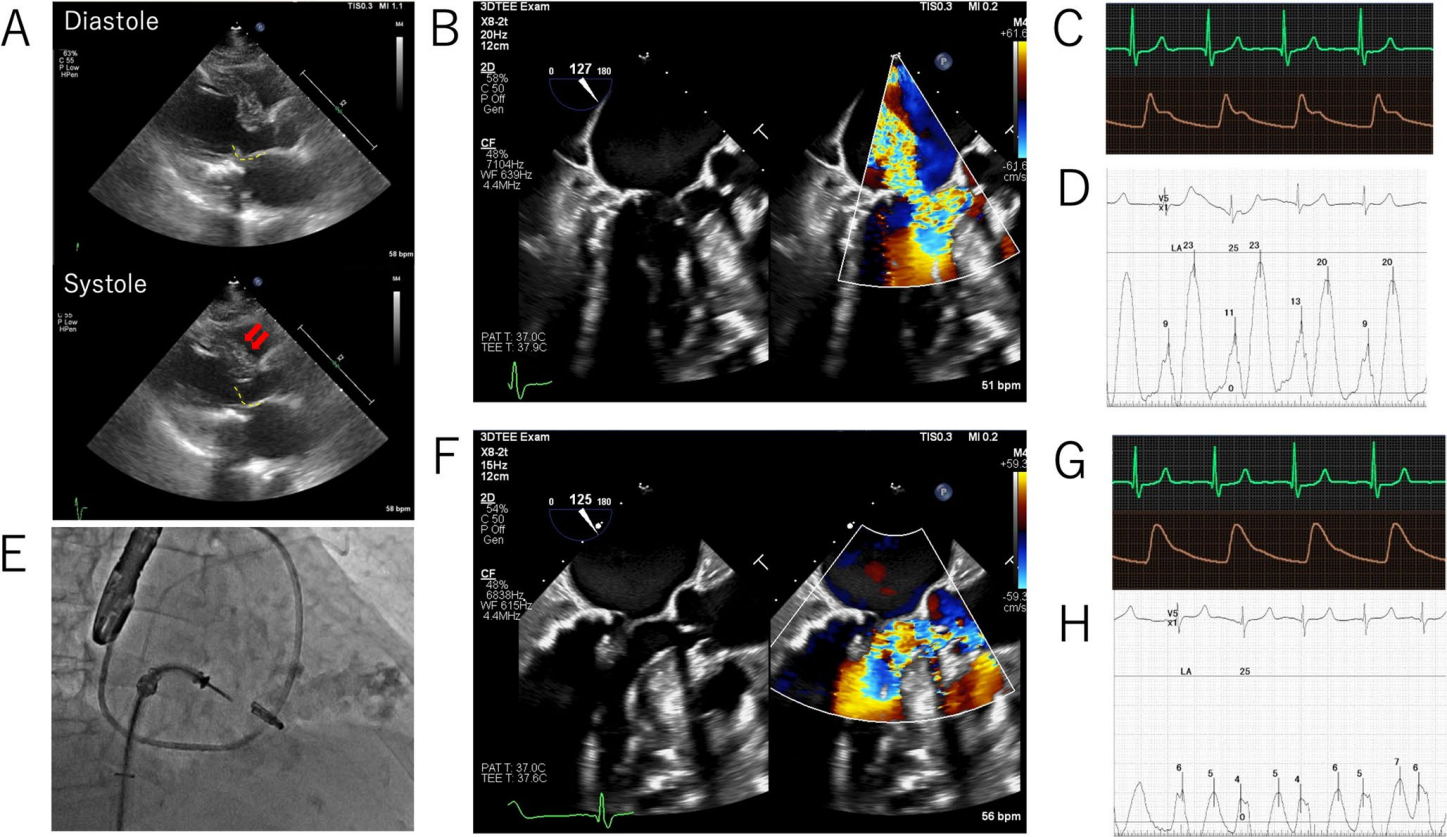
Imaging and hemodynamic changes before and after MitraClip implantation (A) Baseline transthoracic echocardiography showing sigmoid septum (arrow) and systolic anterior motion of the anterior mitral leaflet (yellow dotted line) with severe mitral regurgitation (MR) (B) Baseline transesophageal echocardiography demonstrates severe MR (C, D) Baseline hemodynamics: aortic and left atrial pressure waveform (E) Fluoroscopic image during clip positioning (F) Final transesophageal echocardiography showing trivial MR (G, H) Final hemodynamics: aortic and left atrial pressure waveforms
